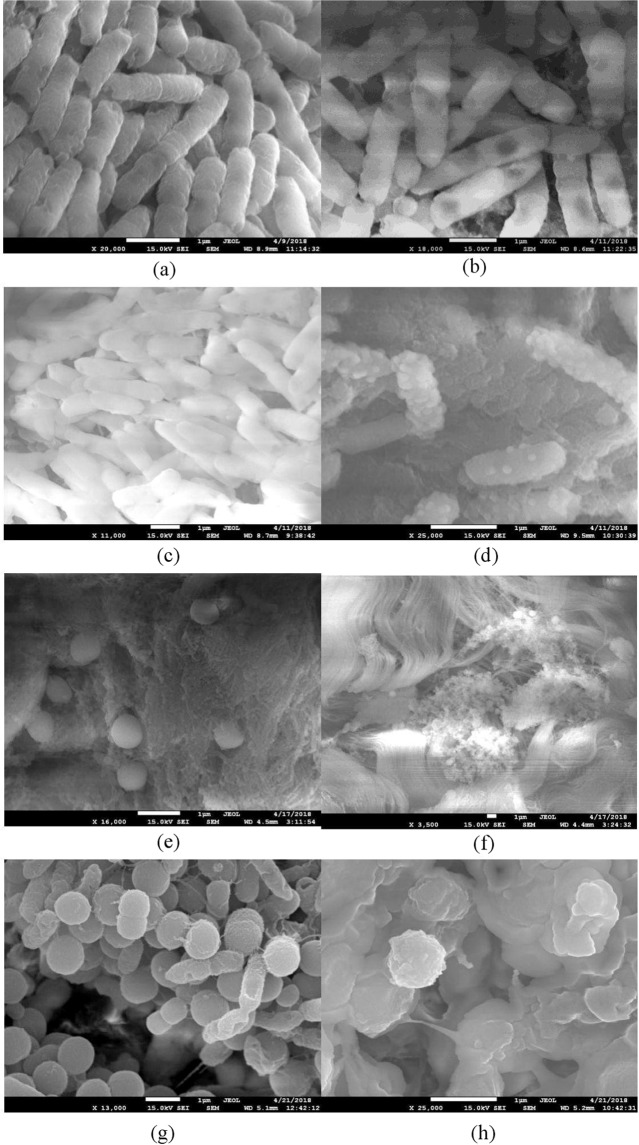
The SEM of *E. coli* and *S. aureus* topography on chicken (a) *E. coli* shape on muscle wash with DI water (b) *E. coli* shape on muscle wash with PAW–ultrasound (c) *E. coli* shape on skin wash with DI water (d) *E. coli* shape on skin wash with PAW–ultrasound (e) *S. aureus* shape on muscle wash with DI water (f) *S. aureus* shape on muscle wash with PAW–ultrasound (g) *S. aureus* shape on skin wash with DI water (h) *S. aureus* shape on skin wash with PAW–ultrasound.
